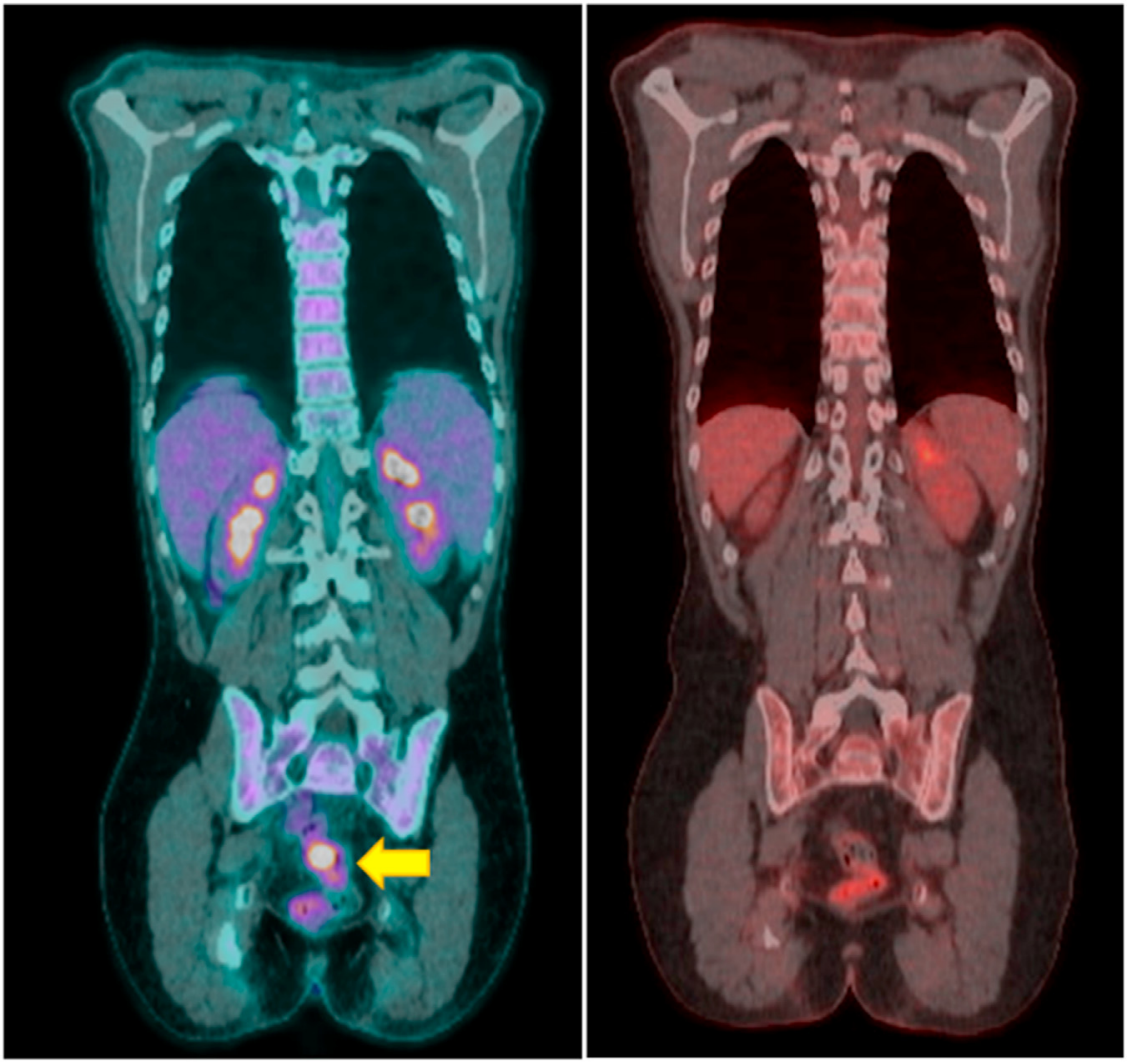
PET-CT scans before and after treatment with rituximab. Before (*left*) and after (*right*) treatment with rituximab and sirolimus shows resolved rectal uptake and decreased spleen size.
